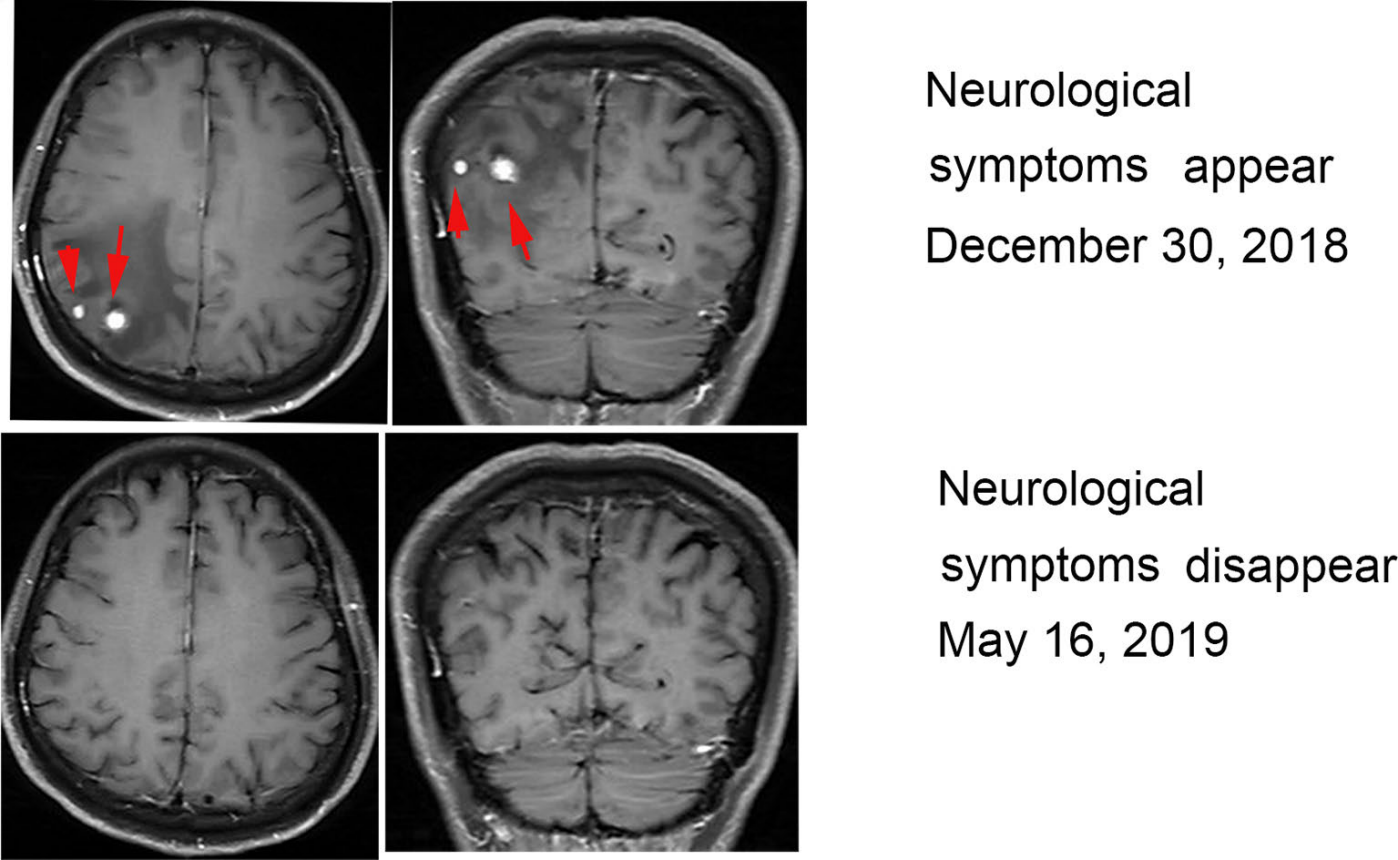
MRI during treatment with nivolumab and CIK cells. MRI revealed two enhancing metastatic lesions within the brain parenchyma 6 months after the initiation of treatment. The brain lesions disappeared after 6 months of continued treatment.
